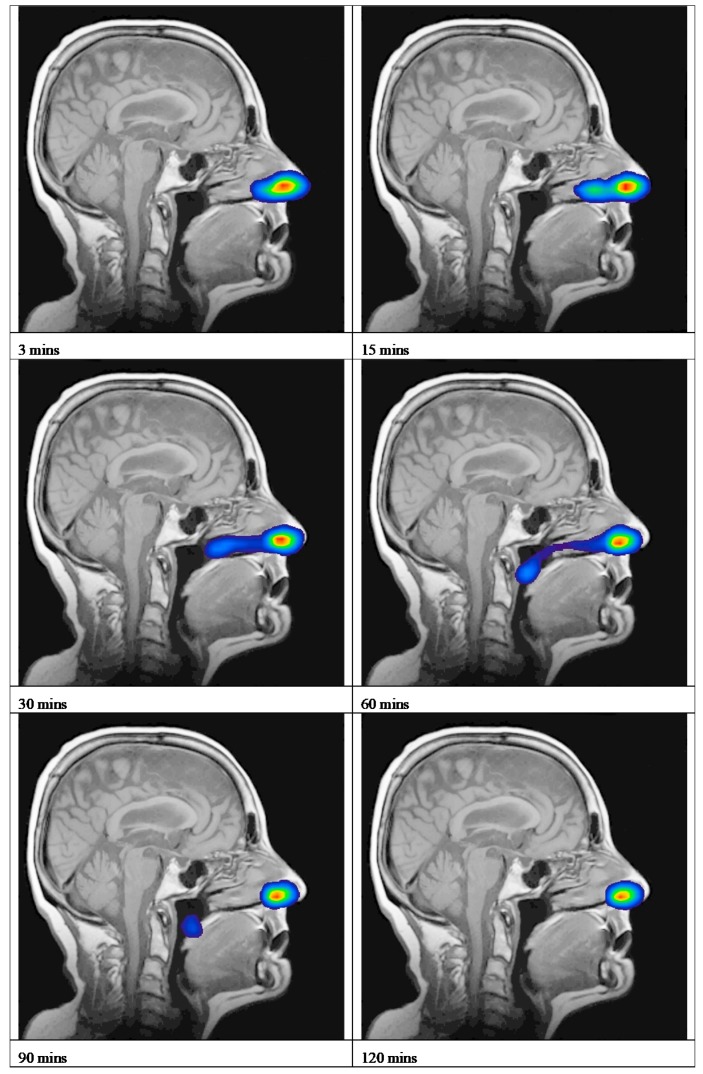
Deposition and clearance of nasal spray formulations delivered using a Rexam SP270 device. Scintigraphic images superimposed upon the MRI sagittal image of Subject 2, showing the clearance of the Tc^99m^-DTPA nasal spray formulation. (The highest levels of activity are shown in red and yellow.).
